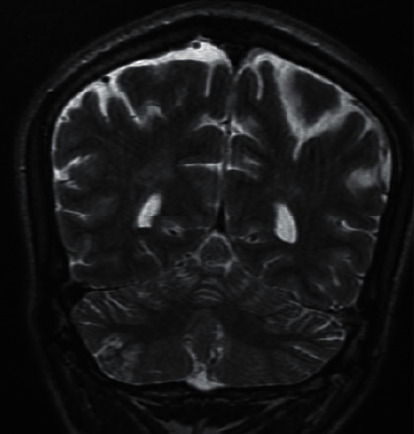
Coronal view of MRI brain showing the right cerebellar infarct.
